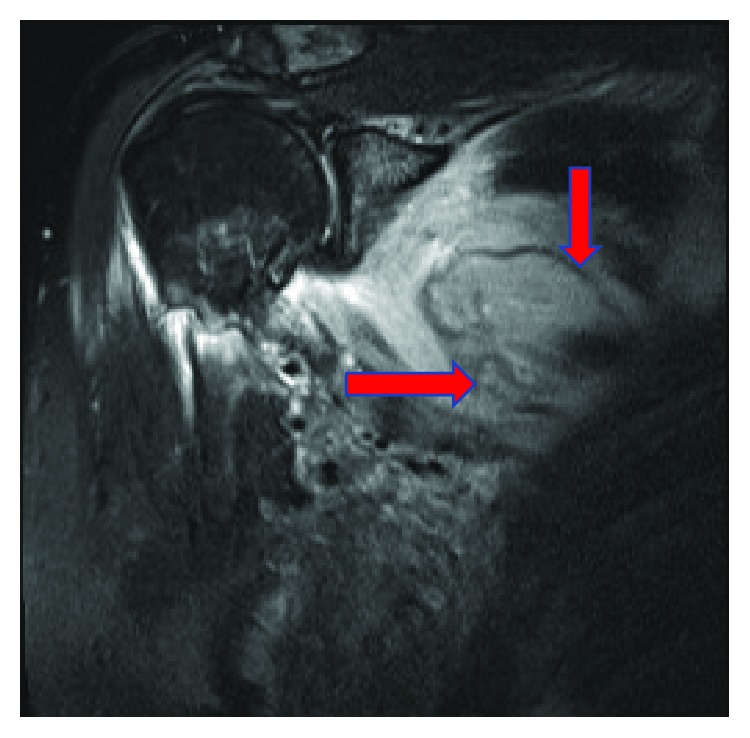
MRI of Rt. shoulder coronal T2 sequence demonstrating well-defined ovoid area of hyperintensity and abscess formation.
